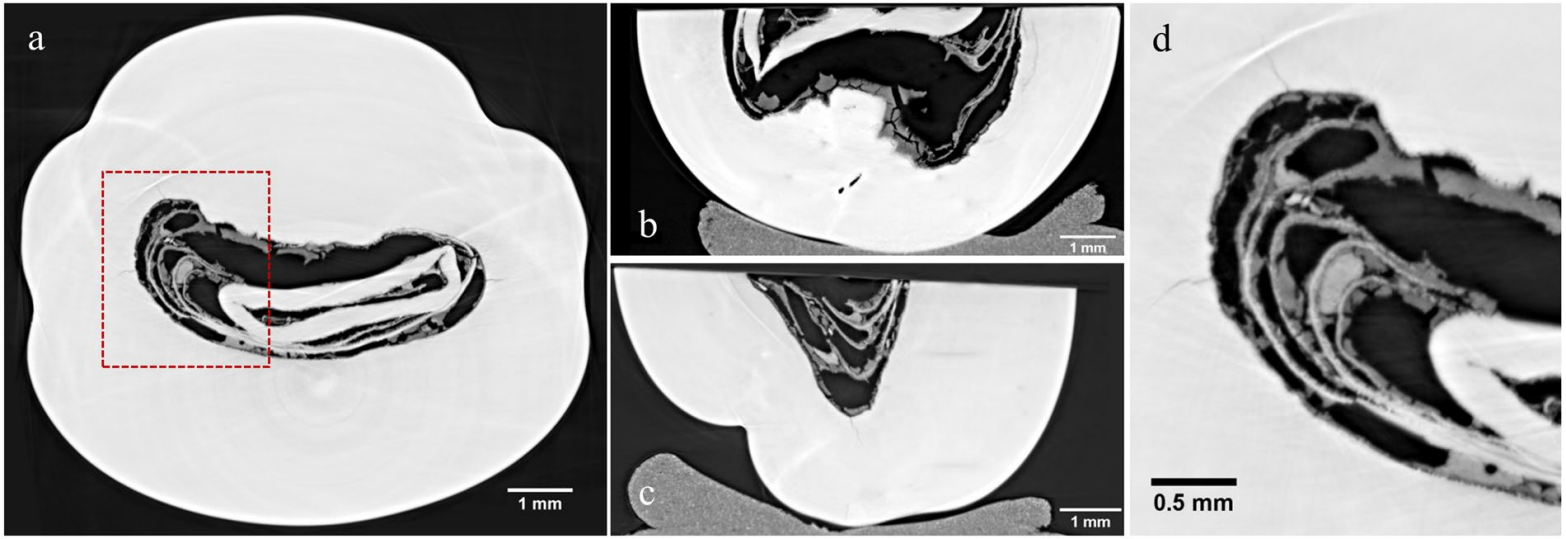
Three orthogonal X-ray PC slices (a–c) and a detail (d) of the slice represented in (a). Small fissures and pearl structures are clearly visible, as well as (in light grey) a carborundum contamination due to the sewing.
